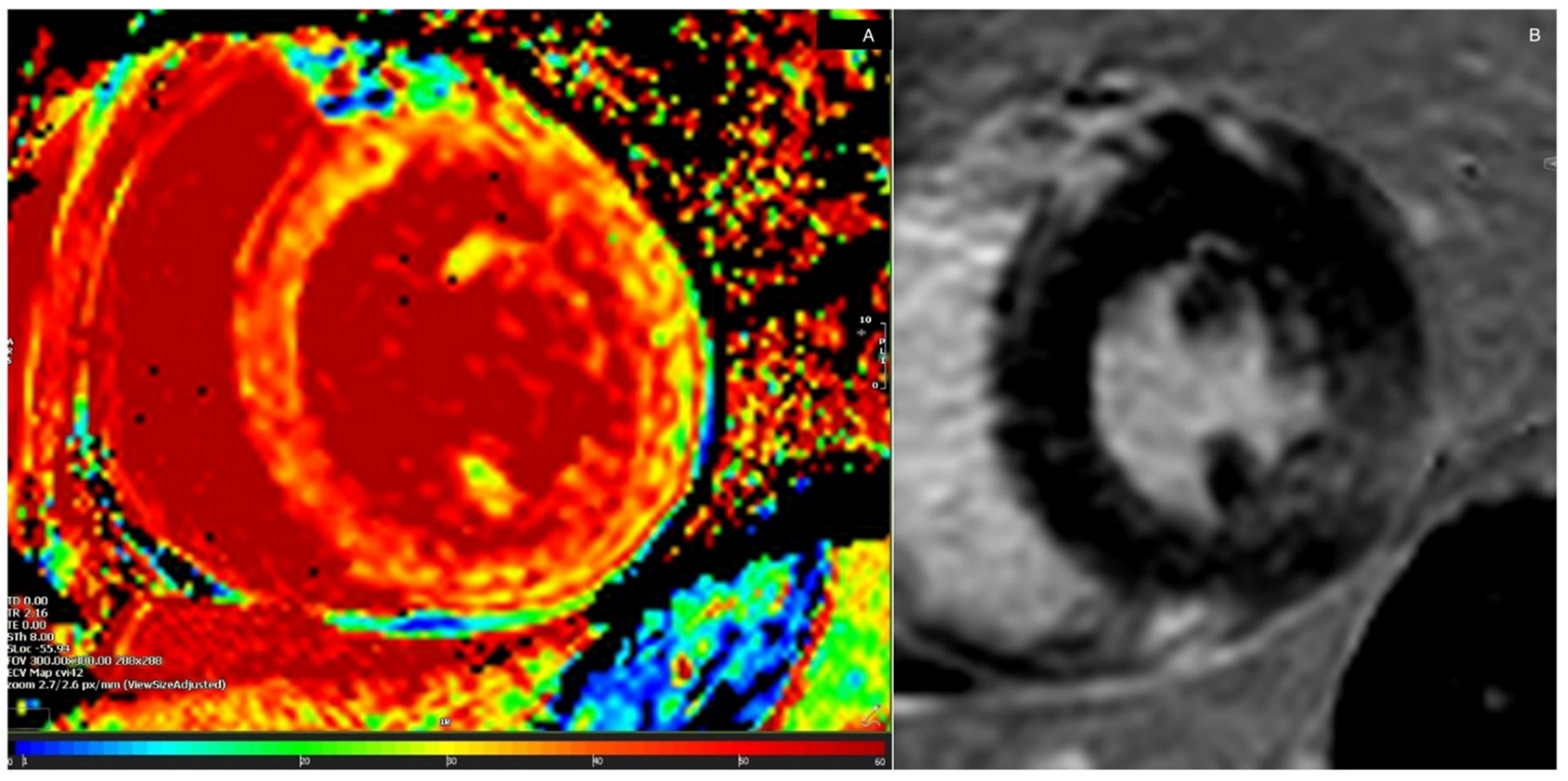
Global extracellular volume by cardiovascular magnetic resonance imaging of Case 1. Pathological values were obtained from the entire left ventricular circumference (A). Focal conspicuities were shown in late gadolinium enhancement (LGE) sequences. This showed inferolateral LGE consistent with regional wall motion abnormalities on echocardiography (B).
